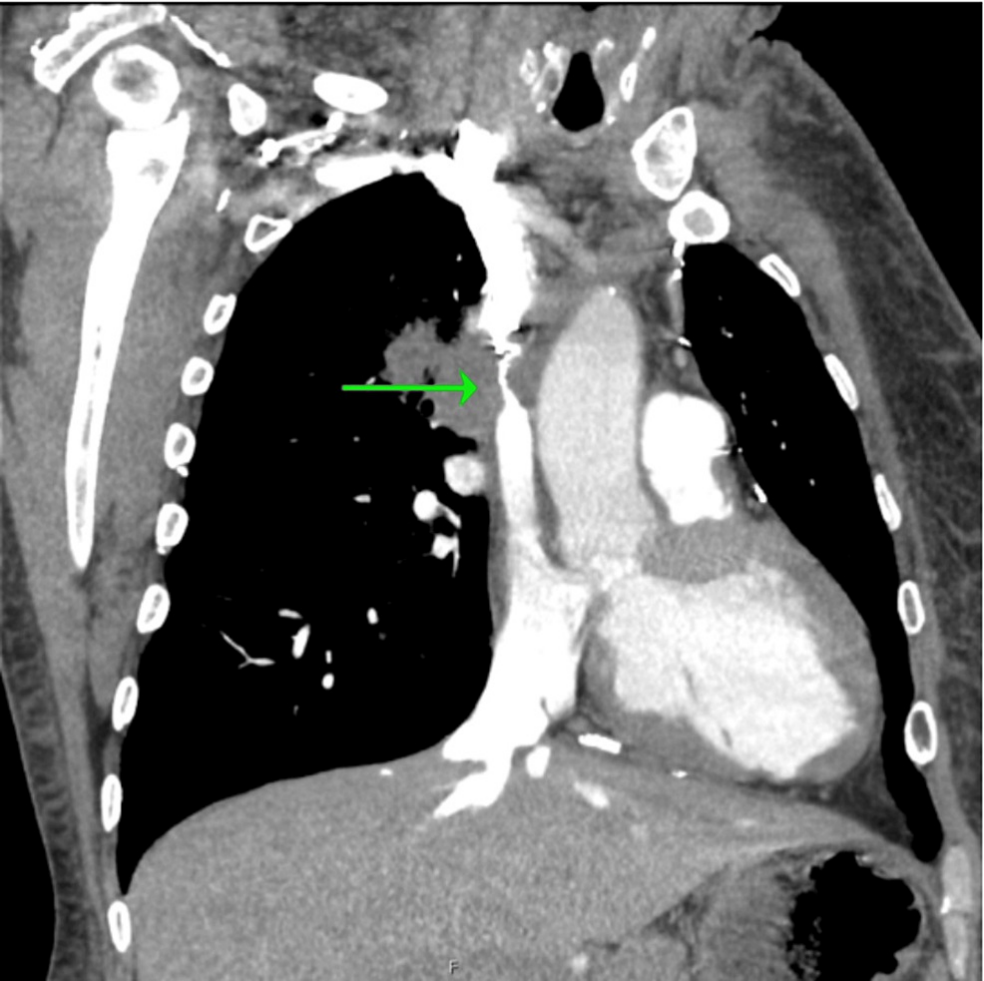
CTPA (coronal view) showing severe short segment narrowing of the SVC from compression by the right suprahilar mass CTPA: computed tomography pulmonary angiography; SVC: superior vena cava
